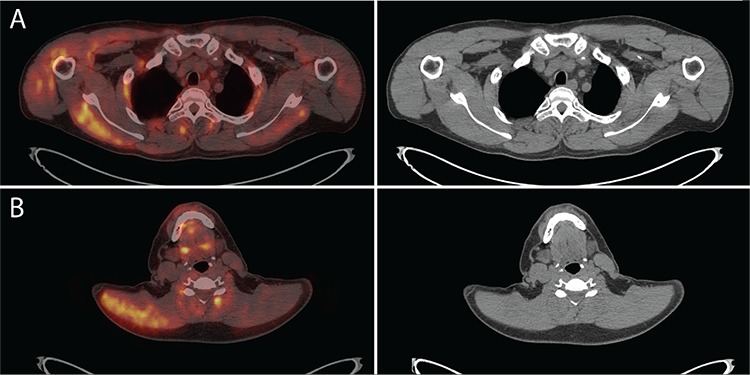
Right biceps muscles showed increased ^18^F-FDG uptake with SUV_max_ 5.0, (A) right shoulder muscles had SUV_max_ 4.0, (B) right trapezius muscle had SUV_max_ 4.1 and right serratus anterior muscle had SUV_max_ 3.8.
